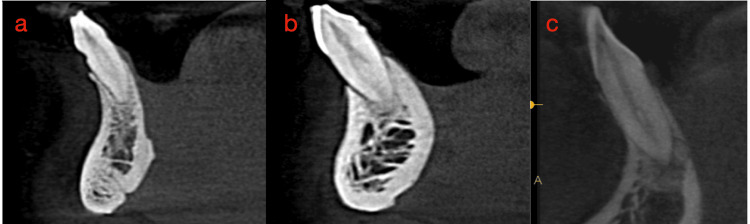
CBCTs depicting two canals and bifurcations of canals. (a) Bifurcation at coronal third. (b) Bifurcation at middle third. (c) Bifurcation at apical third. CBCT - Cone beam computed tomography
